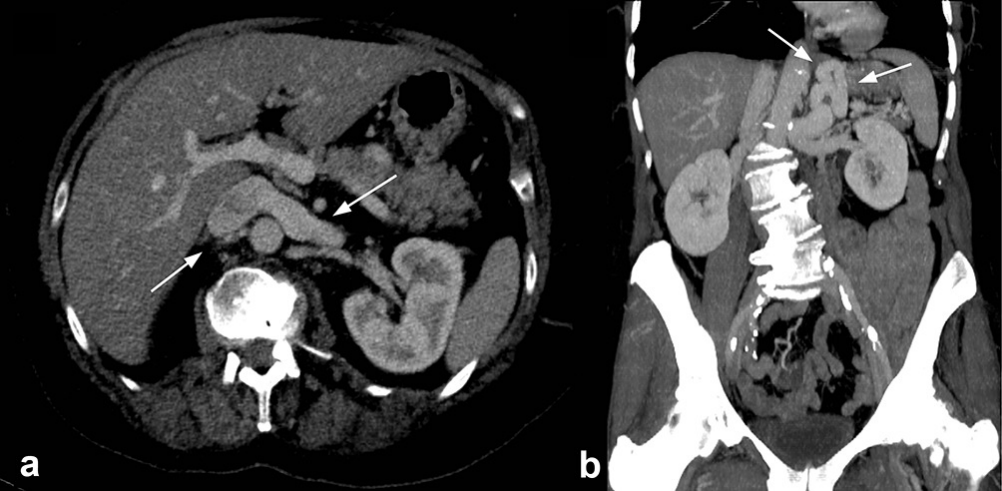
(a) Axial CT image (1 mm, maximum intensity projection) showing an engorged left renal vein posteriorly, relative to the portal vein, due to the congenital extrahepatic portosystemic shunt in a 68-year-old asymptomatic female patient. (b) Coronal reconstructed CT image (5 mm, maximum intensity projection) showing a tortuous and dilated left gastric (shunt) and splenic vein within the left upper abdomen and communicating superior mesenteric vein and portal vein with the left renal vein and inferior vena cava (Supplementary Video 1a,b).
